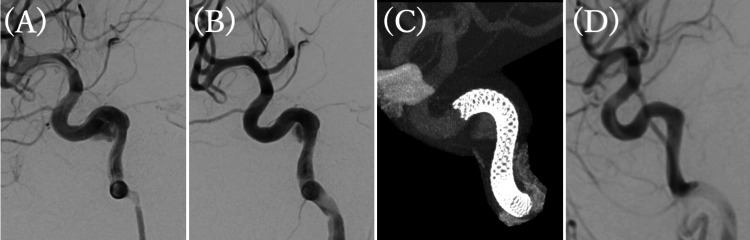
Images during treatment (A) Lateral view of DSA prior to FDS deployment, demonstrating an increase in aneurysm size compared with prior imaging. (B, C) Lateral views of DSA and cone-beam CT after FDS deployment. DSA shows an eclipse sign within the aneurysm, and the FDS extends from the cavernous to the petrous portion, covering the aneurysm. (D) Lateral view of DSA two months post-injury, showing no opacification of the aneurysm, consistent with complete occlusion. DSA, digital subtraction angiography; FDS, flow diverter stents
